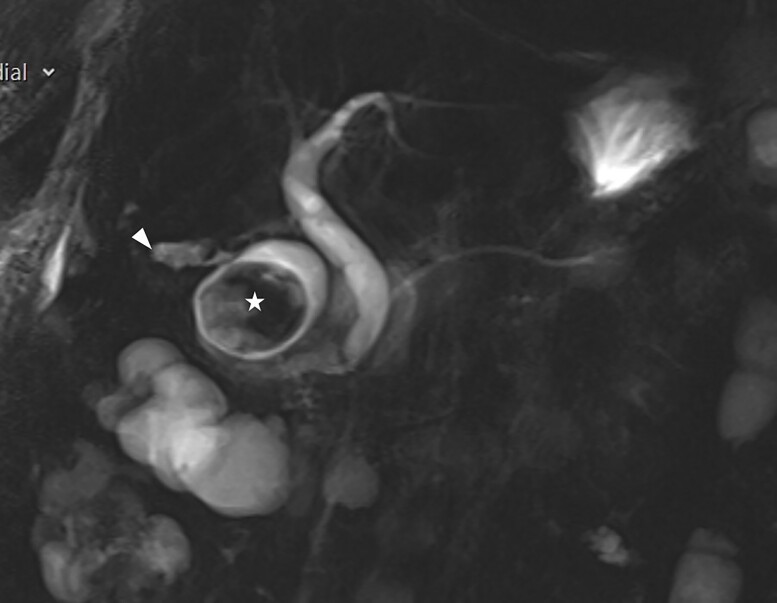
Coronal view of the MRI showing normal biliary tree.
